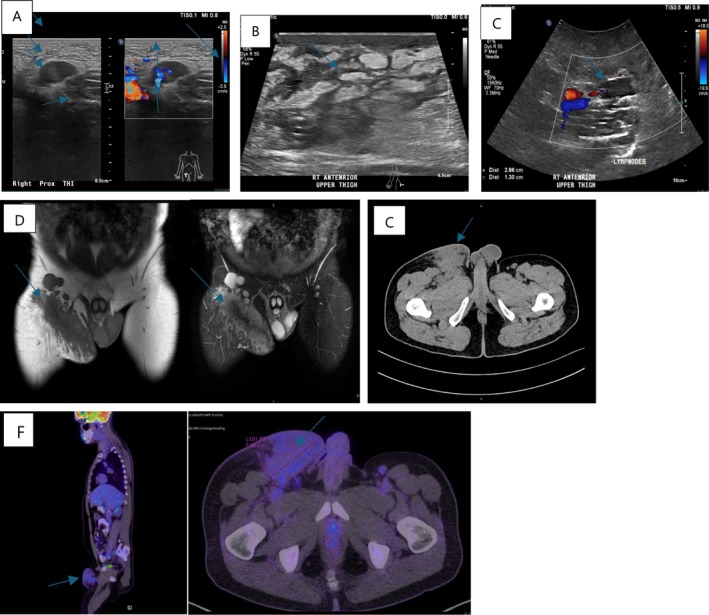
(A) US 2021 Rt anterior upper thigh with doppler. (B) US 2023 Rt anterior upper thigh. (C) US 2023 Rt anterior upper thigh with doppler. (D) MRI, coronal section with and without contrast. (D, E) right thigh raised suspicion for chronic subcutaneous infection. Granulomatous disease with associated lymphadenopathy (blue arrows). (E) CT pelvis, transverse section. (F) PET scan, right upper anterior thigh suggestive of an inflammatory process with low activity, likely infected/inflammatory lymph nodes and reactive single right axillary and left inguinal lymph nodes. (blue arrows).
